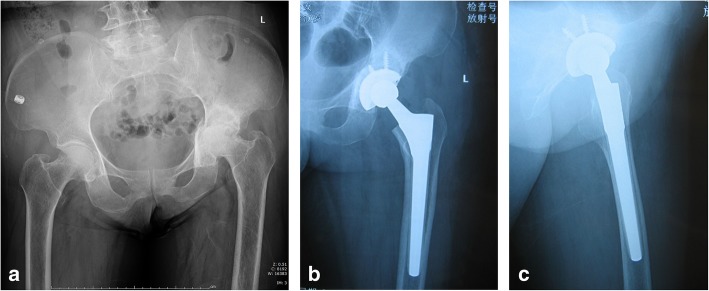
Radiographs of a 70-year-old woman with rheumatoid arthritis of the left hip. a Preoperative radiograph of left hip demonstrates the collapse of the left femoral head with Dorr type C femoral bone. b Immediate postoperative anteroposterior radiographs demonstrate the good canal filling of the Wagner SL stem. c Frog-leg lateral radiograph in left side
